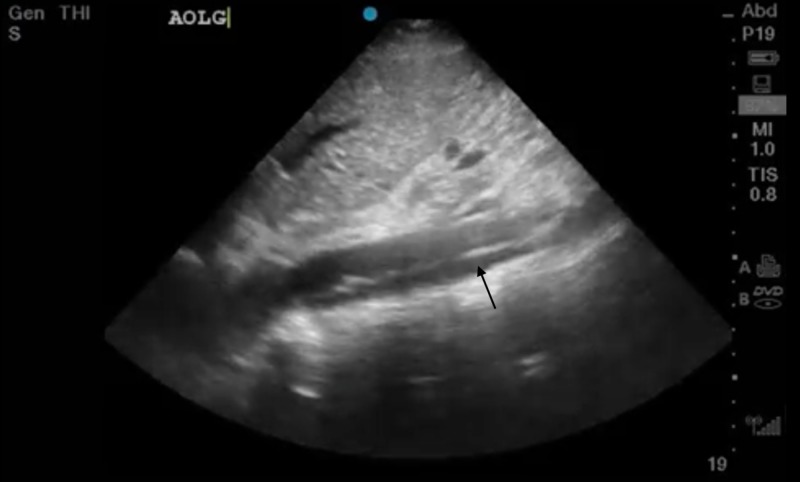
Longitudinal ultrasound image of intimal flap in abdominal aorta
